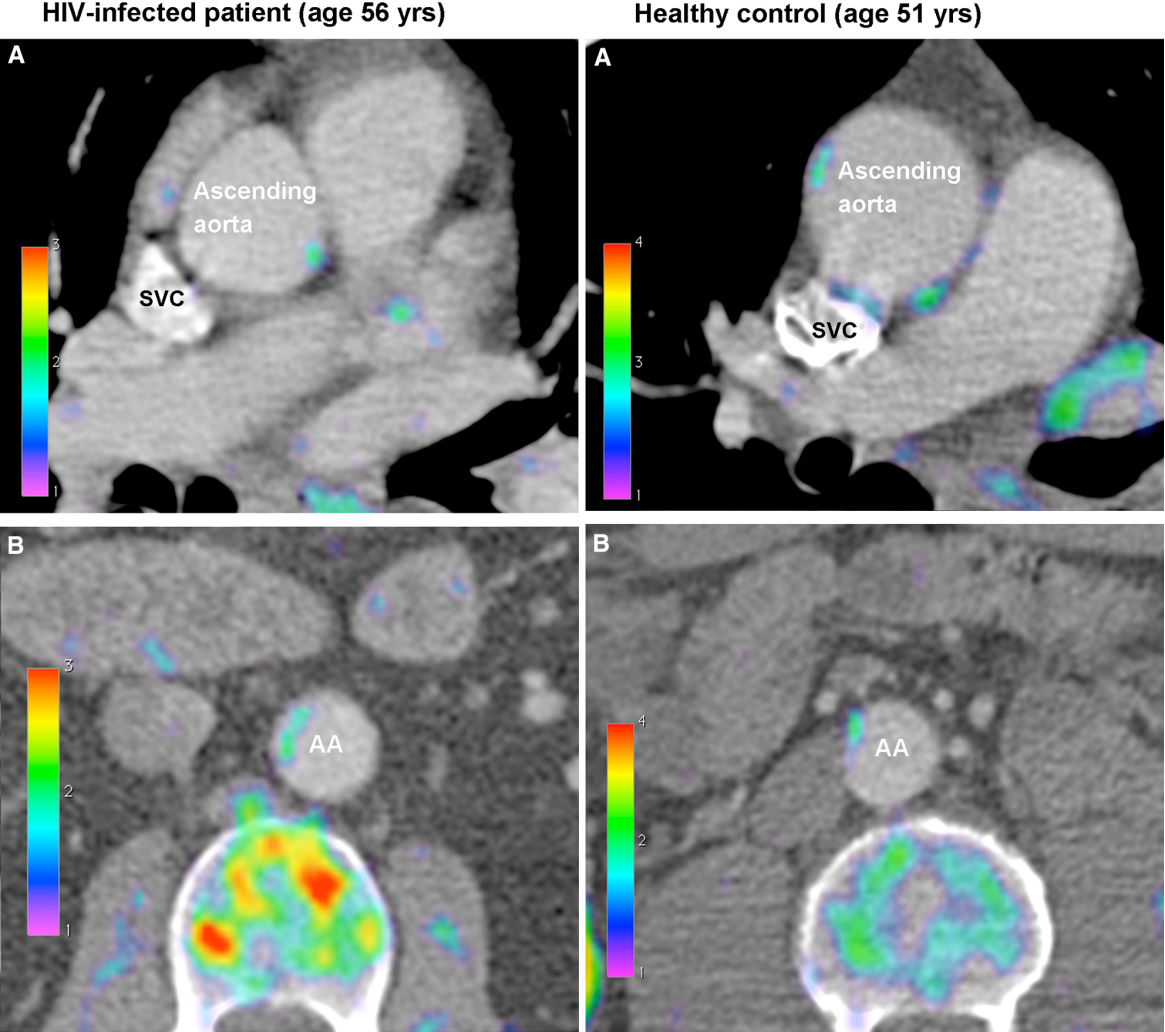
Fusion PET/CT axial images from the ascending (*panel A*) and abdominal aorta (*panel B*) of an HIV-infected patient and a healthy control showing modest FDG uptake in the arterial wall. *AA*, abdominal aorta; *FDG*, fluorodeoxyglucose; *SVC*, superior vena cava
